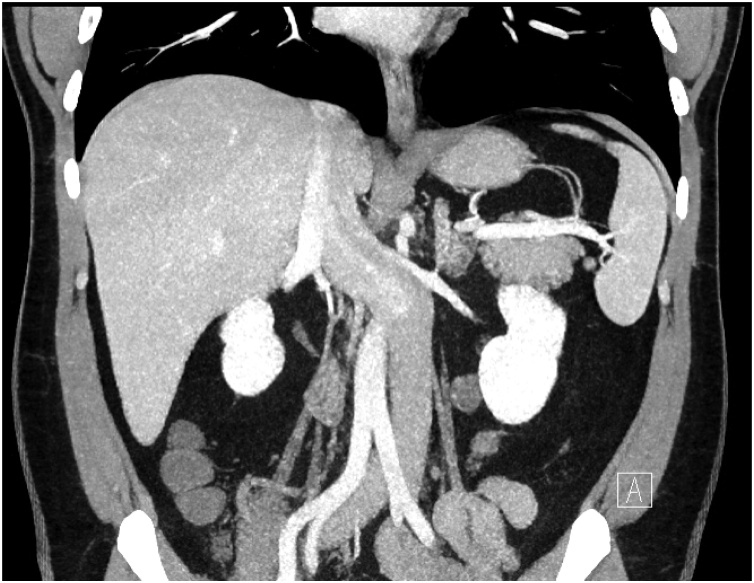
Abdominal CT showing IVC located at the left side of the Aorta.
